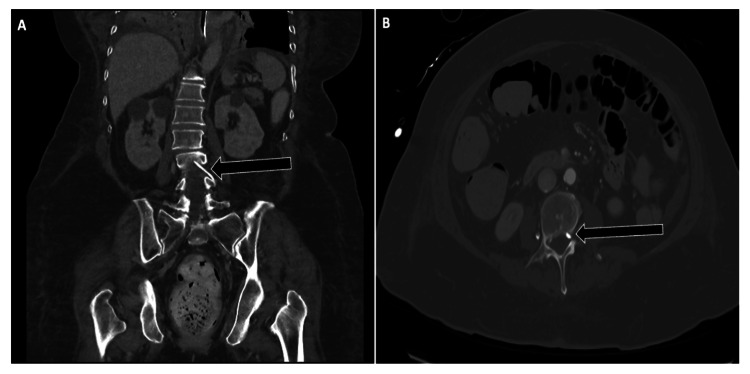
Unusual Femoral Catheter Malposition Into the Spinal Canal Non-contrast CT of the abdomen and pelvis demonstrating malposition of a left femoral venous catheter tracking into the spinal canal via collateral venous pathways.
